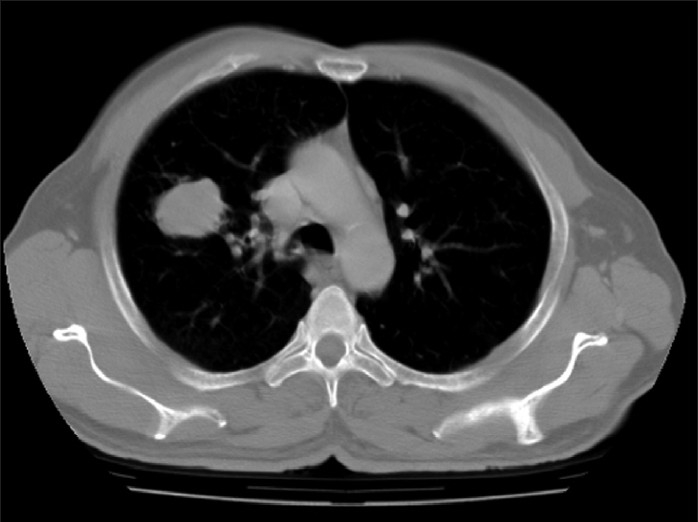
CT scan of chest showing the mass lesion in the anterior segment of right upper lobe with smooth lobulated margins
